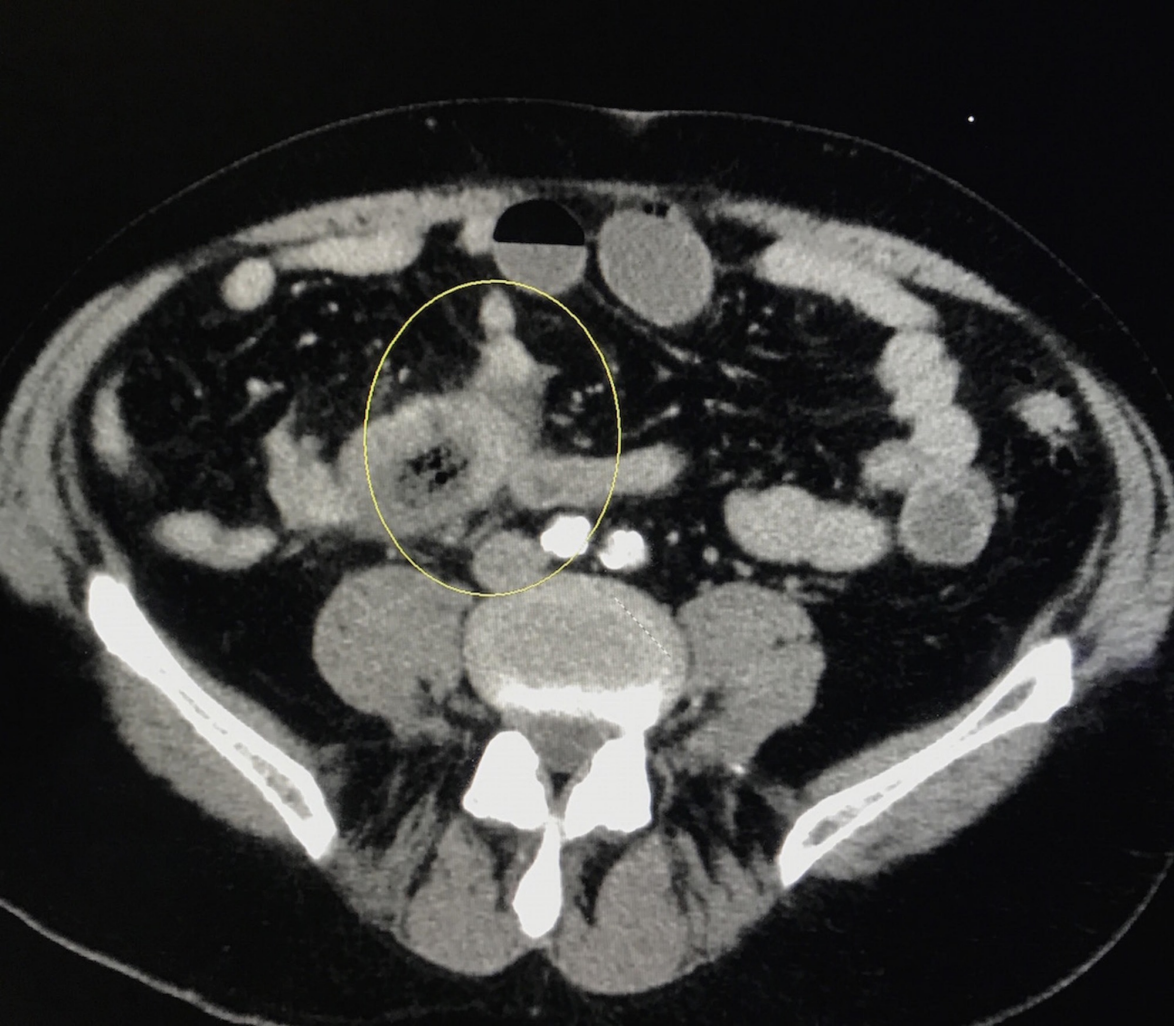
Computed tomography of the abdomen and pelvis demonstrating intermediate grade small bowel obstruction.
